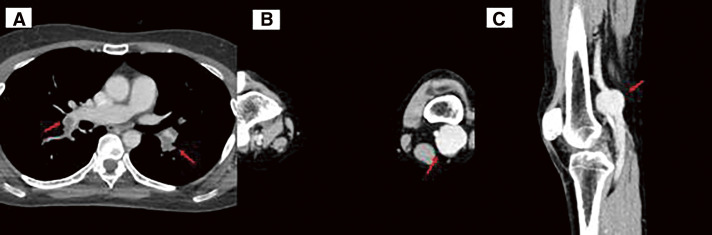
Fig. 1 Preoperative contrast-enhanced CT. (A) CT reveals a submassive PE. The right side is occluded from the superior trunk pulmonary artery bifurcation, and the left side is occluded from the lingular artery bifurcation. (B) CT reveals a saccular PVA. (C) The PVA has a maximum diameter of 32 mm and a length of 30 mm. CT: computed tomography; PE: pulmonary embolism; PVA: popliteal venous aneurysm
